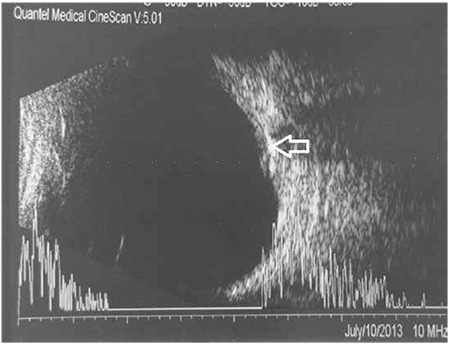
Buried drusen (arrows) have hyperechogenic appearance on B-scan ultrasonography
